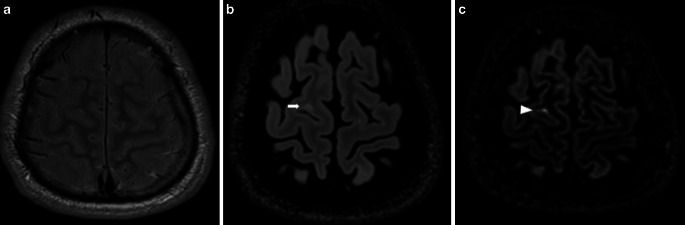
Cortical/juxtacortical lesions in a patient with PPMS. MRI scan in a patient with PPMS. a Proton-density (PD)-weighted image in the axial plane. Nonvisible cortical lesion in the right parietal region. b Fluid attenuated inversion recovery (FLAIR) image, axial plane. A poorly visible cortical lesion in the right parietal region (*white arrow*). c Double inversion recovery (DIR) image of a clearly visible lesion in the same location (*white arrowhead*)
